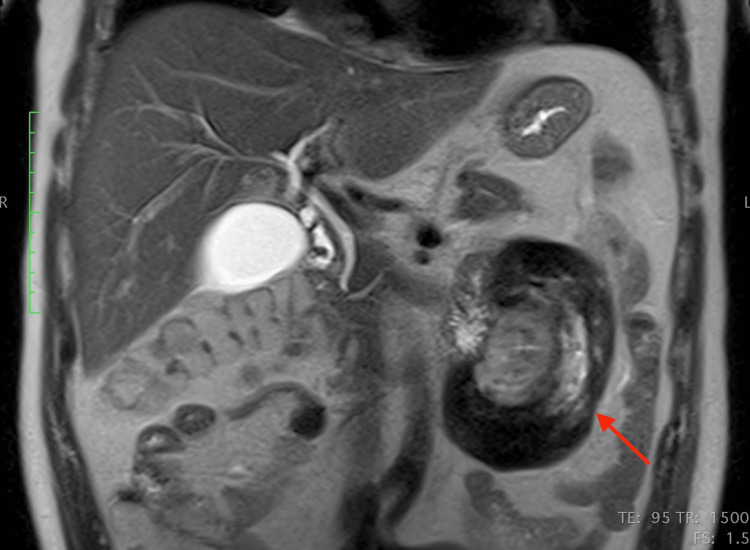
Coronal MRI T2-weighted image MRI: magnetic resonance imaging
